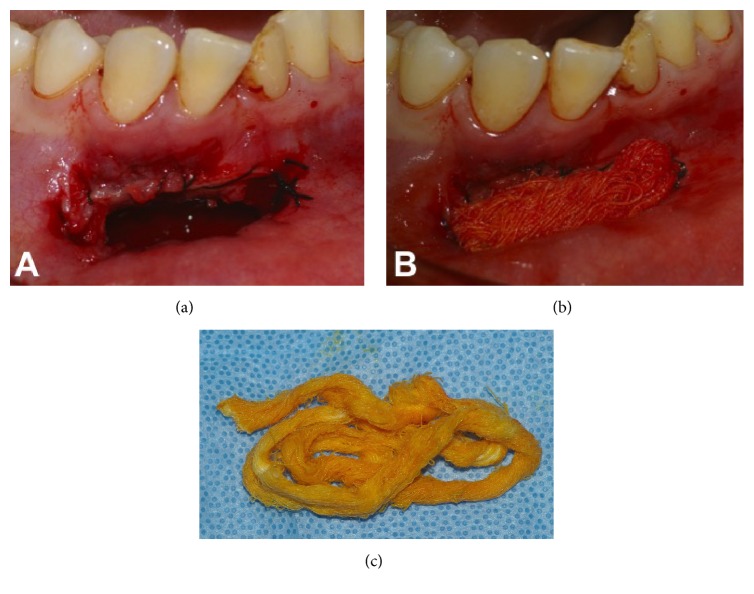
Surgical access through the anterior mandible for decompression of periapical lesion. (a) Cavity after packing (b) with gauze impregnated with antibiotic ointment (c).
